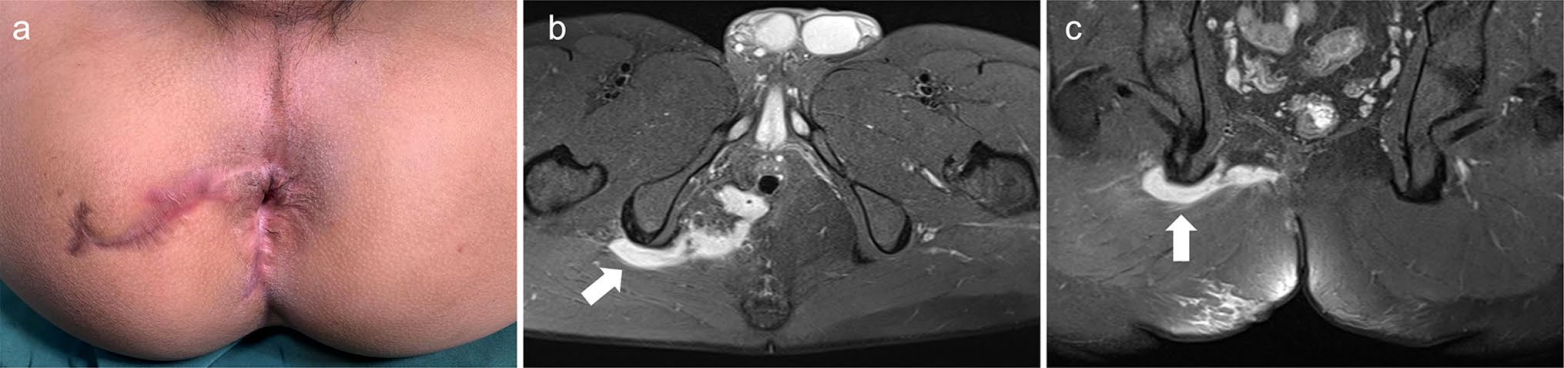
A 28-year-old man with a history of three operations for complex anal fistula presented to our department complaining of perianal discomfort. a Physical examination revealed 2 old surgical scars around the anus. b–c Perianal magnetic resonance imaging (MRI) was used for preoperative diagnosis and showed that the fistula passed through the anal sphincter to the outer lower edge of the right ischial tuberosity (white arrow)
